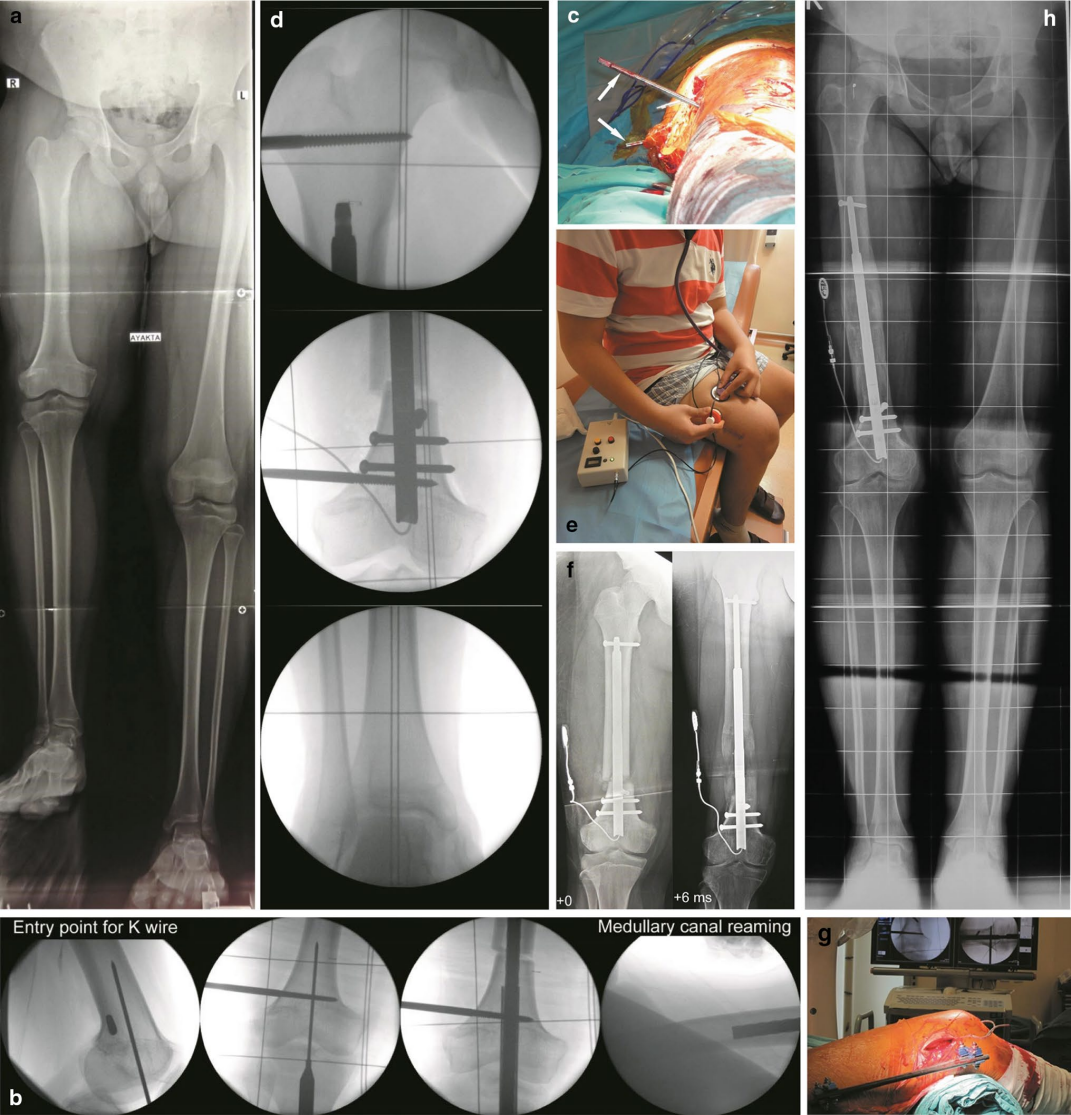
A 14-year-old male with 14 cm of femoral shortening, 6° varus, and 30° rotational deformity was treated with two consecutive retrograde Fitbone applications. a) Preoperative LSR while the leg length differences were compensated for using plates and the patellae were oriented anteriorly. b) The standard entry point of the K-wire for retrograde femoral nailing and medullary canal reaming was used with rigid reamers. The K-wire should be placed exactly as in the preoperative plan for accurate deformity correction. c) A 30° acute rotational correction was completed before reaming the proximal medullary canal. d) Position of the blocking screw and intraoperative alignment control using a grid plate (see the text). e) Distraction using activation of the transmitter head and the control electronics. f) The x-rays in the early postoperative period and 6 months after 8 cm of distraction. g) The Fitbone was replaced with a new one after the first distraction period, and the femur was distracted a further 6 cm. A temporary external fixator was used intraoperatively to protect the distraction site. h) LSR after 14 cm of lengthening and deformity correction
